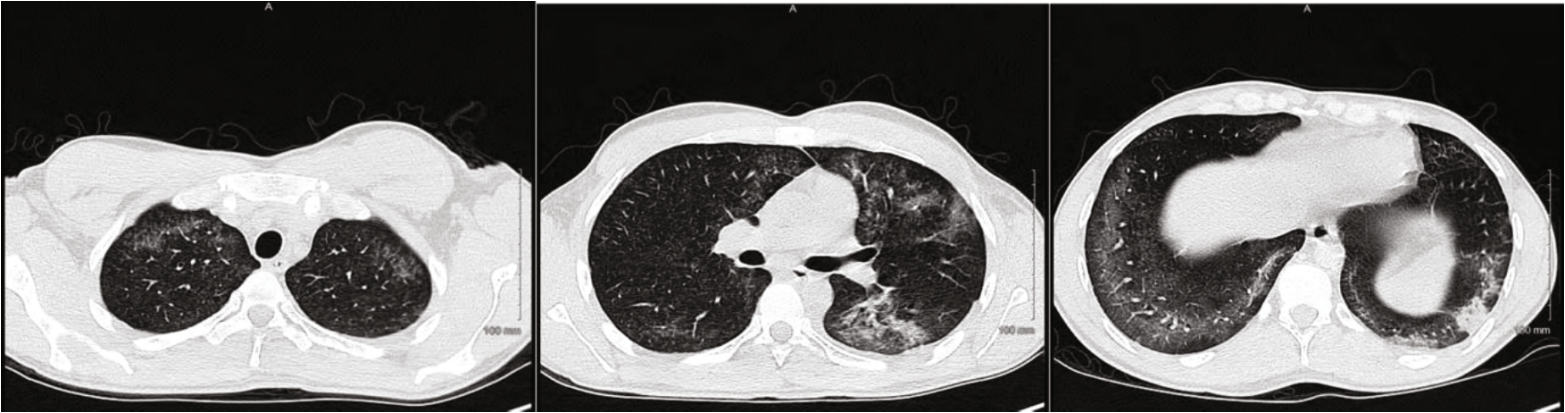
Chest CT showing bilateral diffuse infiltrates.
